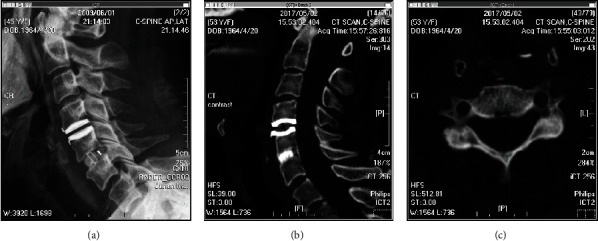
Sample patient showing anterior bone loss (ABL). (a) Lateral radiograph of a 45-year-old woman taken one month after cervical disc arthroplasty with Bryan Disc over the C4-C5 level. (b, c) Computed tomography scan of the same patient 8 years postoperation found grade 2 ABL. Note the preserved cortex and bone density.
